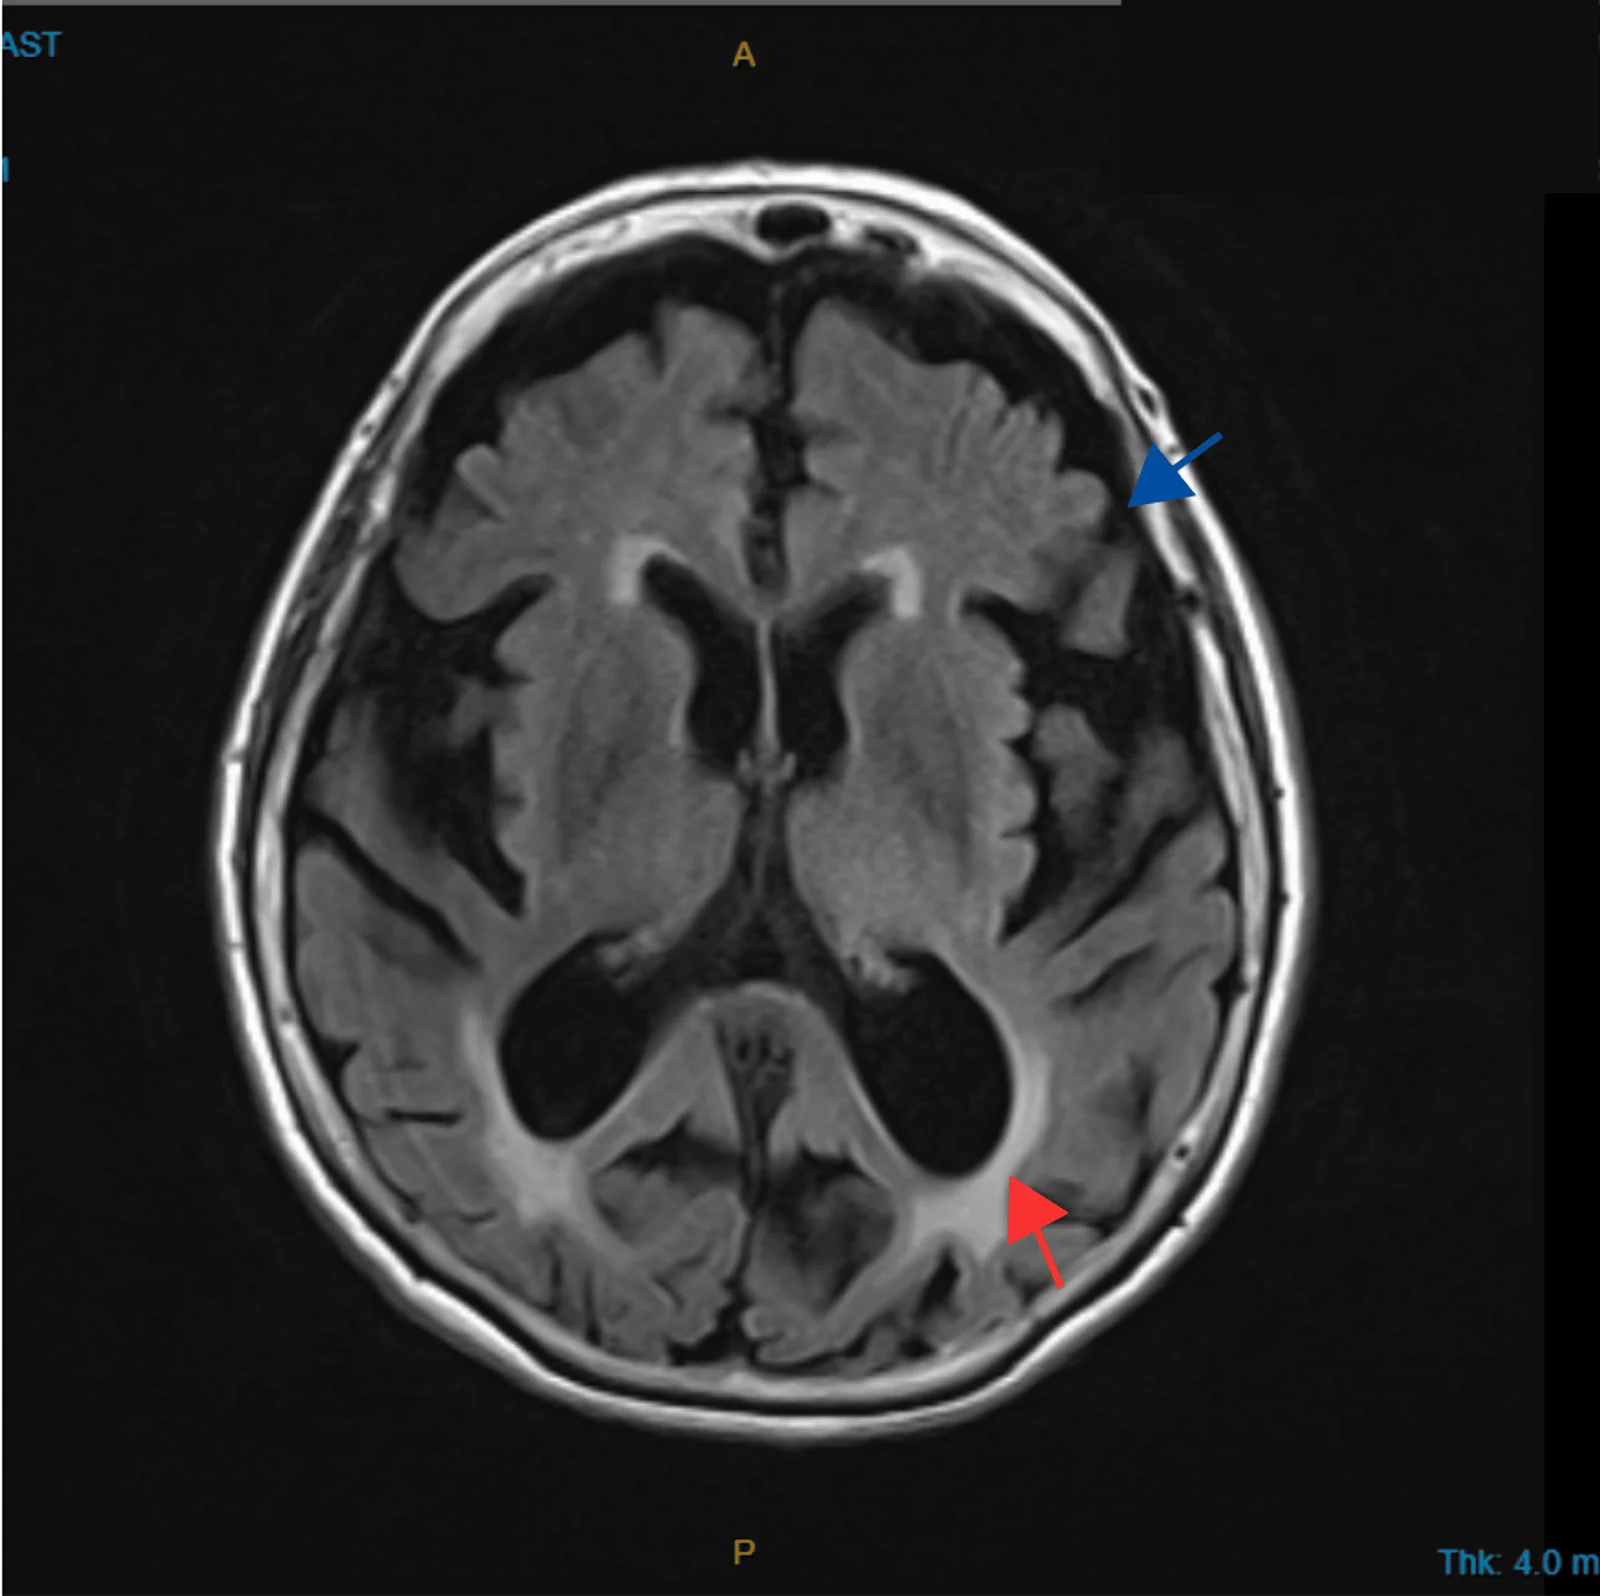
MRI brain: axial T2 T2-FLAIR (fluid-attenuated inversion recovery) Brain MRI demonstrating severe diffuse cerebral volume loss (blue arrow) and moderate-to-severe nonspecific periventricular and subcortical white matter signal abnormalities (red arrow), consistent with chronic microvascular ischemic changes.
